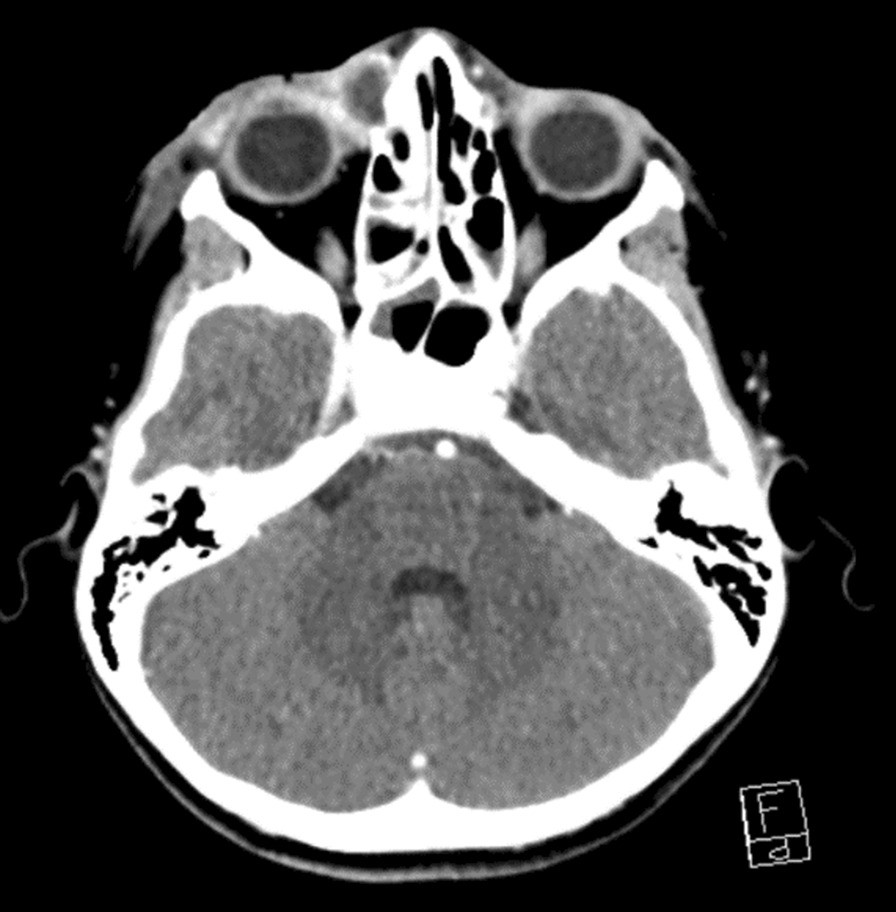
CT scan showing a right preseptal infiltration attributed to cellulitis with an ipsilateral well-delimited collection measuring 11 × 18 × 13 mm. Multiple bilateral cervical lymphadenopathies are also present and concordant with acute EBV infection
